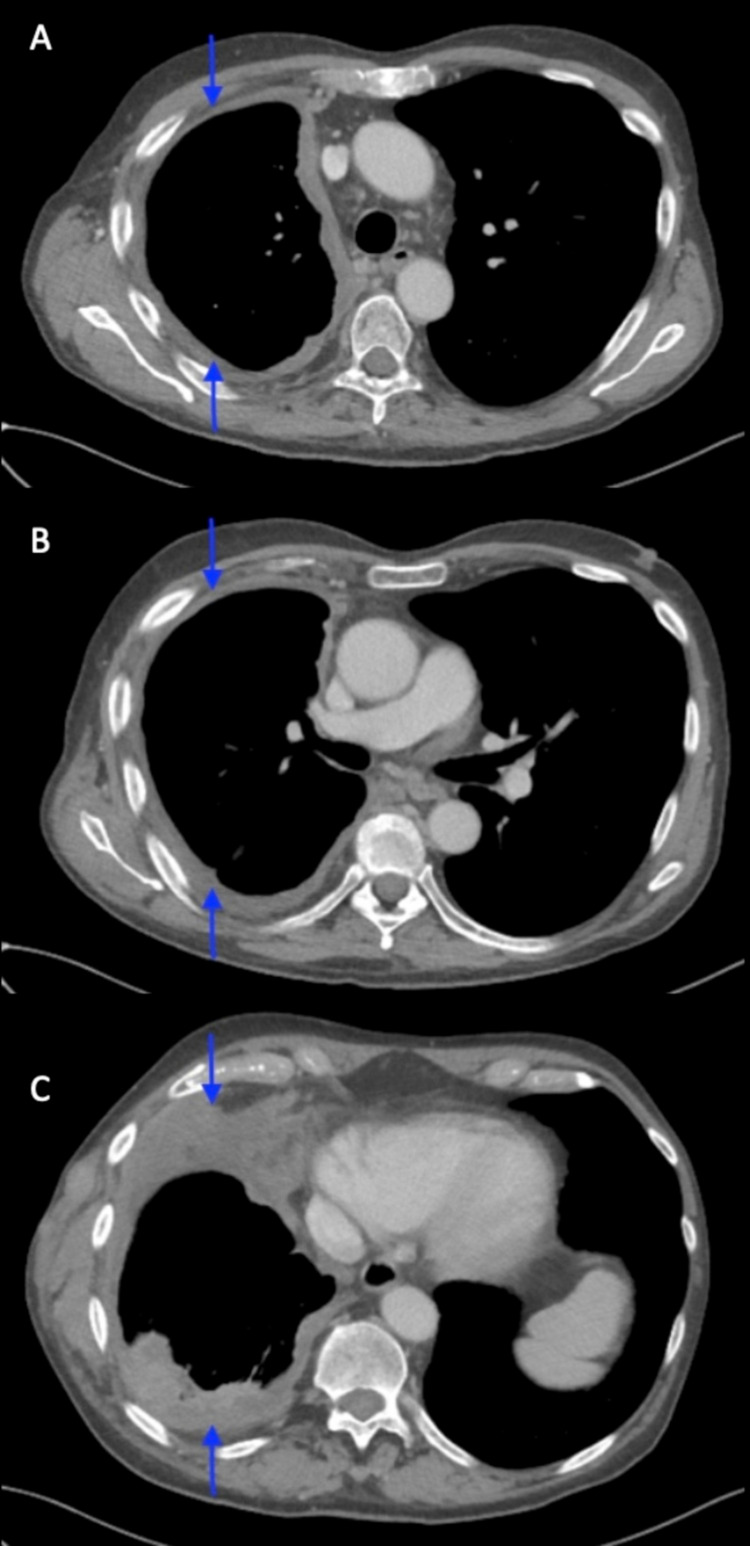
CT Chest 5 months after completion of RT, after 12 cycles of pembrolizumab. Approximated aerated right lung is 90%. A) Right pleural based confluent mass has substantially improved to 12x1mm along the lateral superior mediastinum. B) Compression of right mainstem bronchus resolved. C) Lateral chest wall mass decreased to 23x80mm. Reduction in extent of tumor mass.
